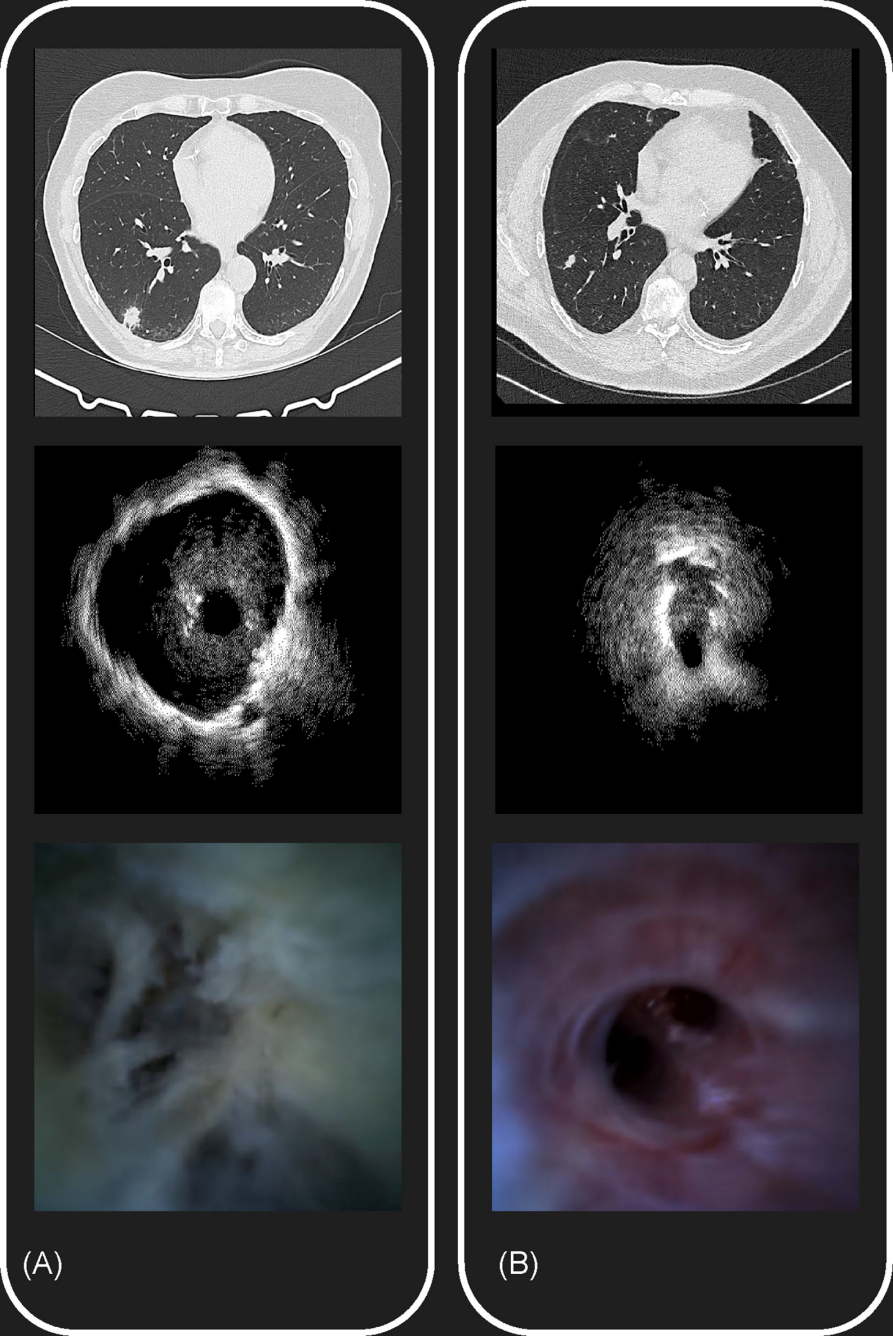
CT, r‐EBUS and Iriscope appearance of a 19 mm subpleural lung cancer and a 7 mm benign lesion. (A) Upper panel: CT scan image of a right lower lobe nodule; middle panel: Centred r‐EBUS image of the nodule; Lower panel: Tumoral aspect with ‘fish flesh’ appearance on Iriscope (final diagnosis: Adenocarcinoma). (B) Upper panel: CT scan image of a right lower lobe nodule; middle panel: Tangential r‐EBUS image of the nodule; lower panel: Normal parenchyma aspect on Iriscope (final diagnosis: Infection).
